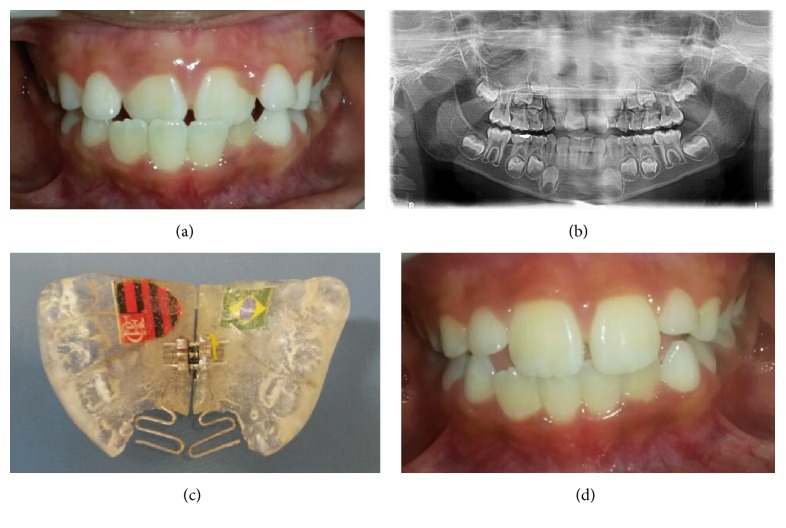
Case  2. (a) Anterior dental crossbite in the two upper central incisors. (b) Panoramic radiograph showing no abnormality. (c) Removable orthodontic appliance with a protrusion spring for each incisor in anterior crossbite. (d) Anterior crossbite correction after 5 months.
